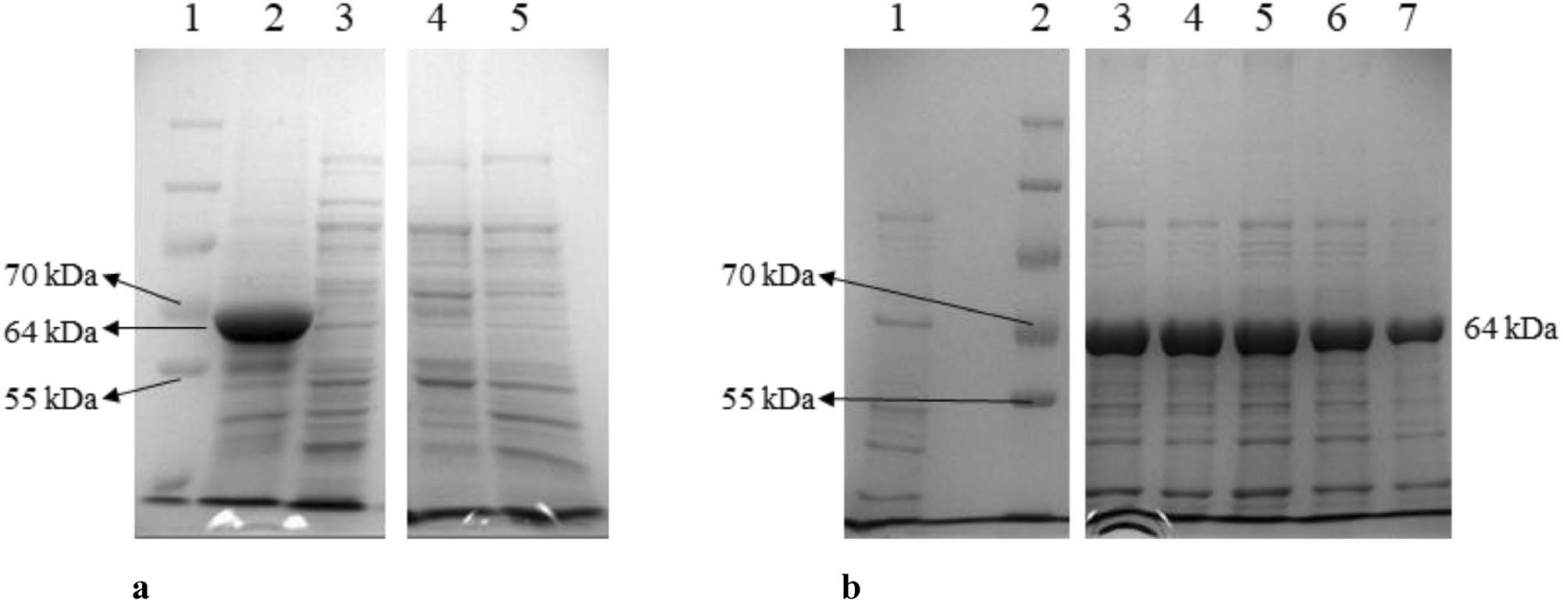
Analysis of inclusion bodies by SDS-PAGE. (a) Lane 1: Protein markers (from top to bottom: 250, 130, 100, 70, 55 kDa) Lanes 2 and 3: Inclusion bodies isolated from the insoluble and soluble fractions of ultrasonicated *E. coli* pET28-LipI.3_KE38 cells, respectively. Lanes 4 and 5: Inclusion bodies isolated from the insoluble and soluble fractions of ultrasonicated *E. coli* pET28 cells which were used as control, respectively. Only relevant lanes on a single gel (Supplementary Fig. S1a) were shown. (b) Analysis of purified inclusion bodies from *E. coli* BL21(DE3) pET28a-LipI.3_KE38 cells (see text for details). Lane 1: sample taken just before induction, Lane 2: Protein marker (from top to bottom: 250, 130, 100, 70, 55, 35 kDa), Lanes 3–7: samples taken every 2 h after IPTG induction. Only relevant lanes on a single gel (Supplementary Fig. S1b).
